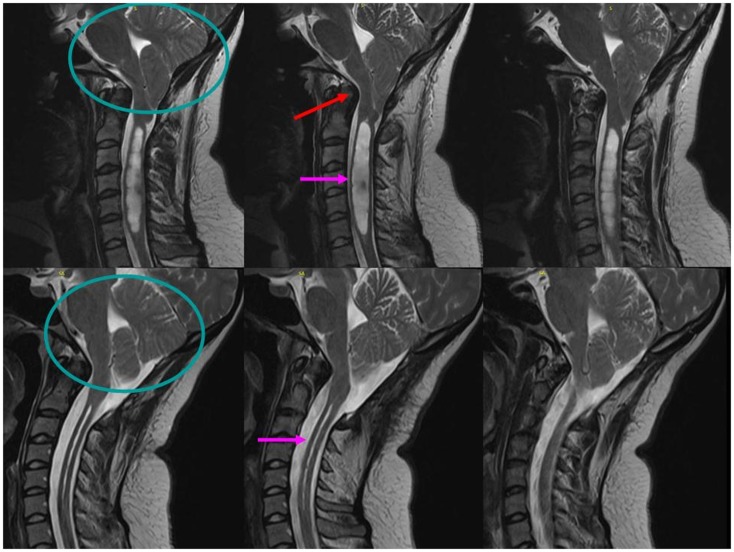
Sagittal T2-weighted MRI scans of the cervical spine demonstrate significant posterior fossa volume reduction (circle) with basilar invagination (red arrow) and a Chiari 1 malformation with 2.2-cm cerebellar tonsillar protrusion below the foramen magnum (circle), resulting in pontomedullary compression and a 1.2-cm maximal diameter cervical cord syrinx (pink arrow, top). Following suboccipital craniectomy and C1–2 laminectomy with duraplasty surgery, the post-operative scans show an increased posterior fossa volume (circle) with adequate decompression of the brainstem and cervical syrinx to a maximal diameter of 12 mm (pink arrow, bottom).
